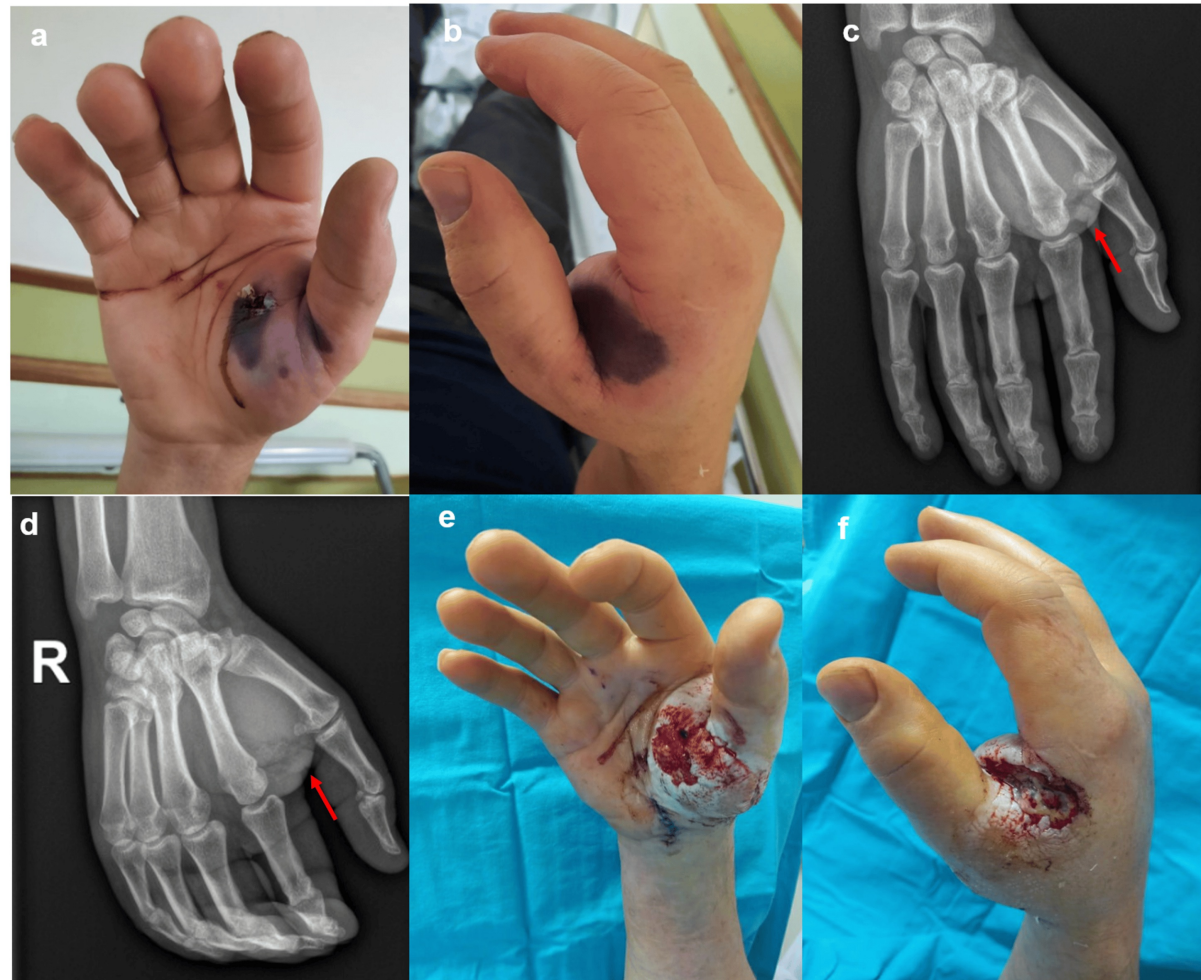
Case 2 (a, b) Admission clinical presentation showing a pinpoint wound with brownish fluid drainage and skin compromise in the thenar region. (c, d) Admission X-ray of the right hand revealing soft tissue edema and subcutaneous emphysema (arrow). The injected material is radiolucent and therefore cannot be seen. (e, f) Later clinical presentation after surgical debridement and wound dressing care, showing lesions in the thenar region that required skin grafting.
